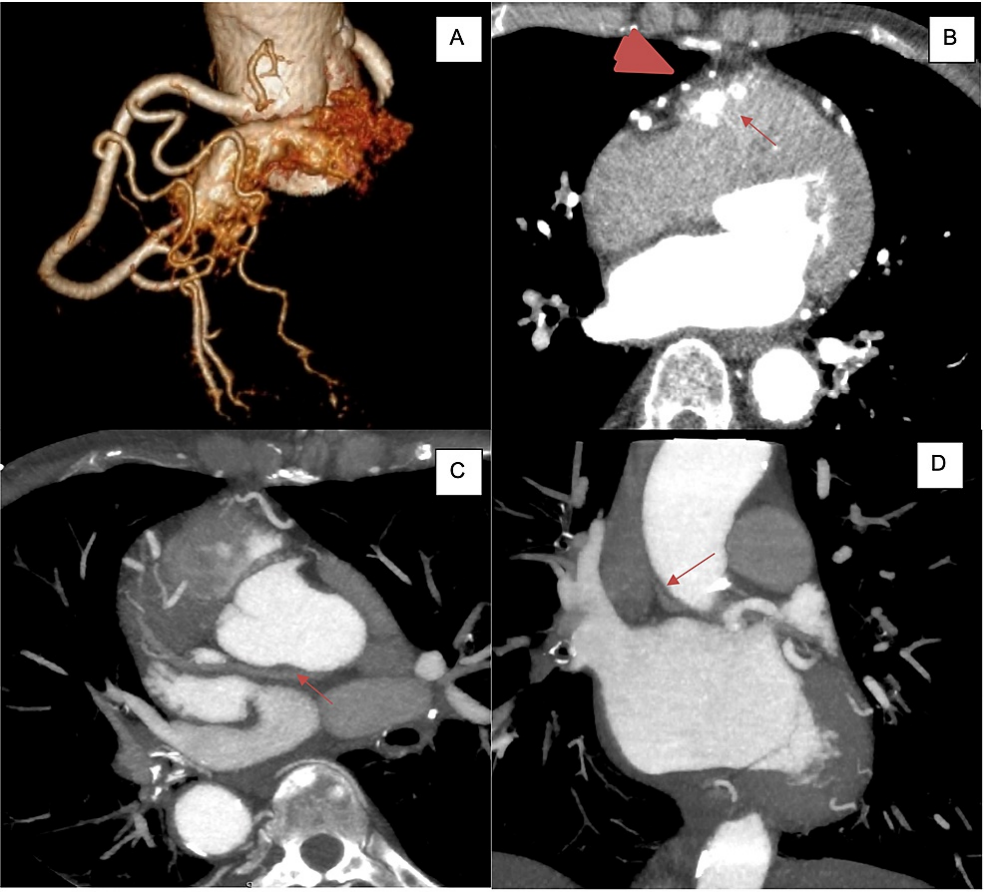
Cardiac computed tomography images. (A) Three-dimensional rendered cardiac computed tomographic image showing a coronary-cameral fistula (part of the right ventricular outflow tract is shown for depiction). (B) Cardiac computed tomography imaging in the axial plane showing multiple arterial branches off the right coronary artery (RCA) (arrowhead) draining into the right ventricular outflow tract (arrow). (C) Axial reformatted computed tomography revealing the course of anomalous coronary vein (originating from the confluence of arterial branches off the RCA and coursing posterior to the aorta). (D) Coronal reformatted images showing the drainage of the anomalous coronary vein into the superior vena cava.
